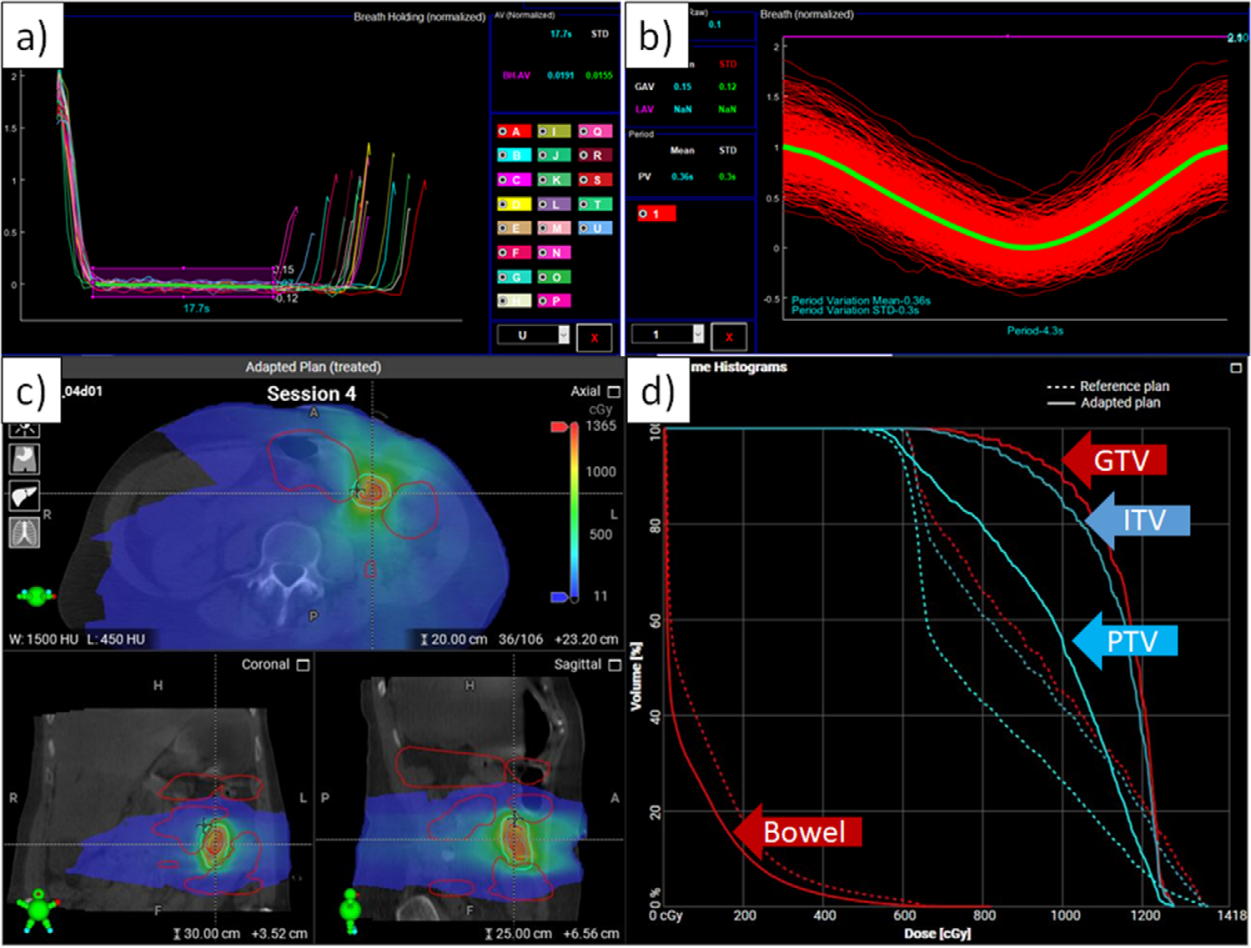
Example of respiratory trace analyses: (a) breath holds of P1 and (b) aligned (red curves) and average (yellow curve) free‐breathing traces of P3; (c) dose map on cone‐beam computed tomography (CBCT) and (d) dose volume histogram of an adapted plan for P1 are presented (dotted lines from the reference plan and solid lines from the adapted plan)
